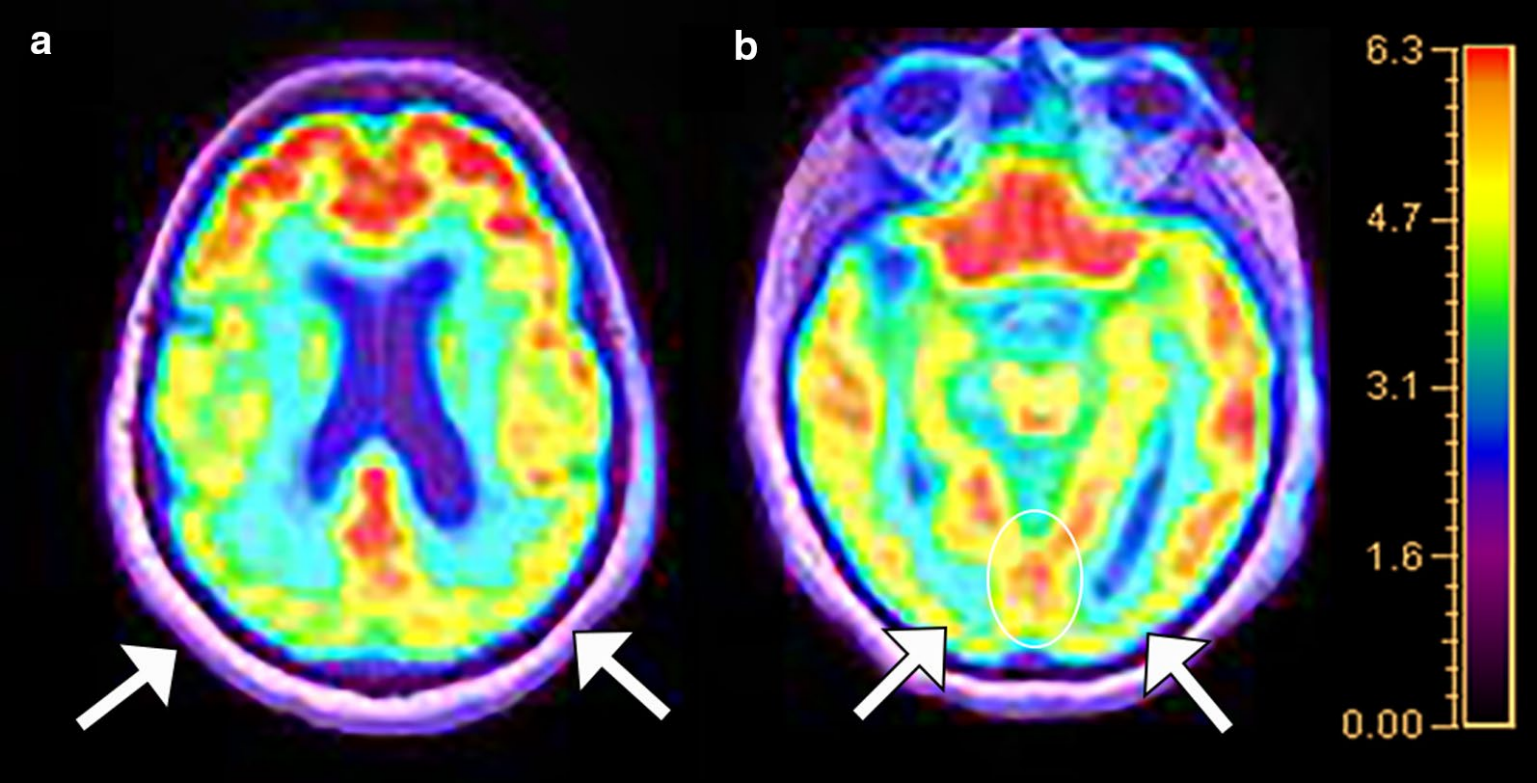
A 76-year-old woman with history of memory loss. Fluorodeoxyglucose PET/MRI (a) demonstrates hypometabolism of the parietal lobes (arrows), precuneus, and PCC as well as the visual association centers in the lateral occipital lobes (arrows in b). Metabolic activity is preserved in the medial occipital lobes (“occipital tunnel sign,” white oval in b). Pattern of hypometabolism is most suggestive of posterior cortical atrophy
